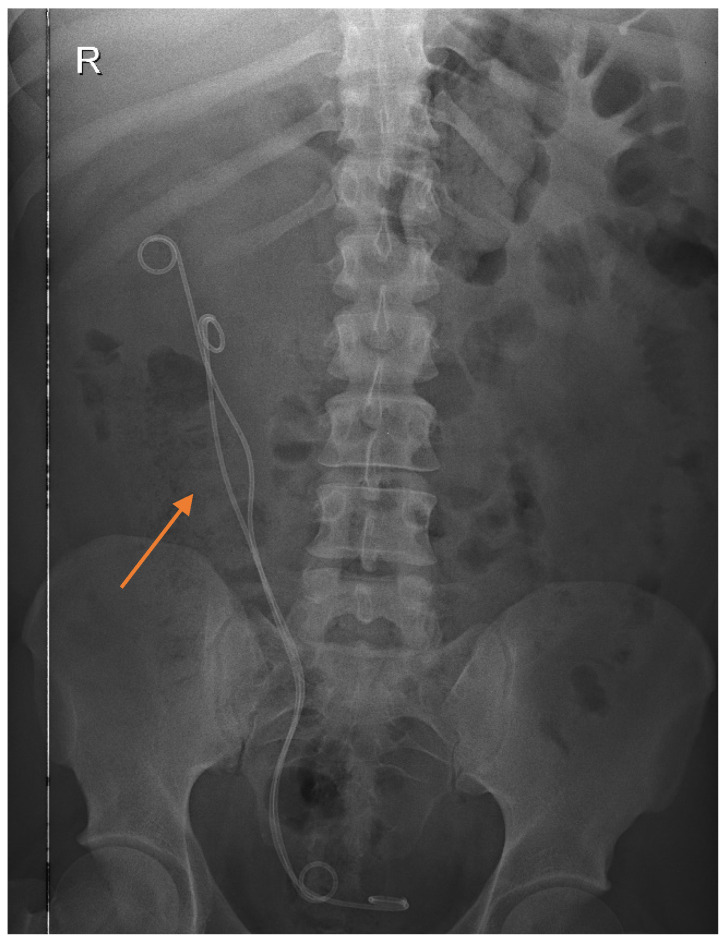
Postoperative radiograph of the abdomen and pelvis following definitive surgical reconstruction (U-trans-U ureteroureterostomy): two double-J ureteral stents, both draining through a single right ureteral orifice into the urinary bladder. The use of dual stenting was required intraoperatively to guide the termino-lateral anastomosis and to ensure patency of both ureteral segments. The anastomosis zone between the two ureters; medially and laterally, in the iliac portion, thus explaining how postoperatively we have two ureters, U trans U (orange arrow).
